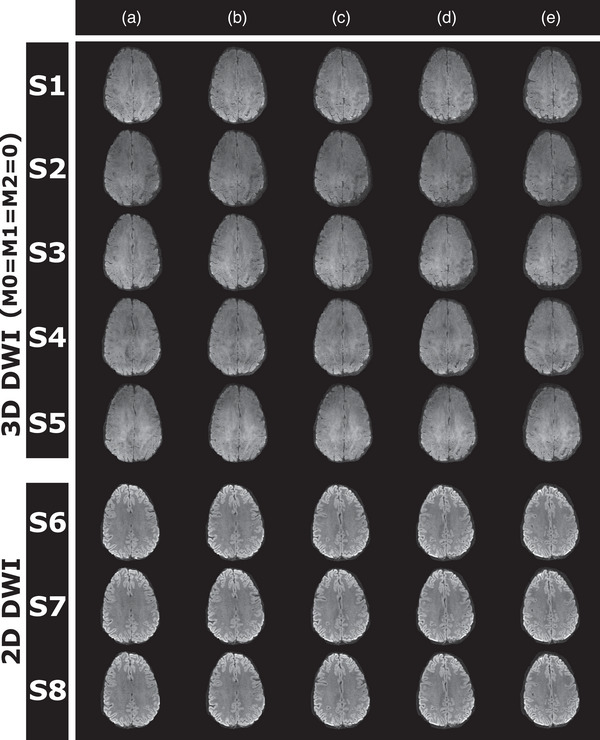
Example trace diffusion‐weighted slices (a–e) of five consecutive 3D‐DWI scans (S1–S5) and three consecutive 2D‐DWI scans (S6–S8) performed in one subject.
